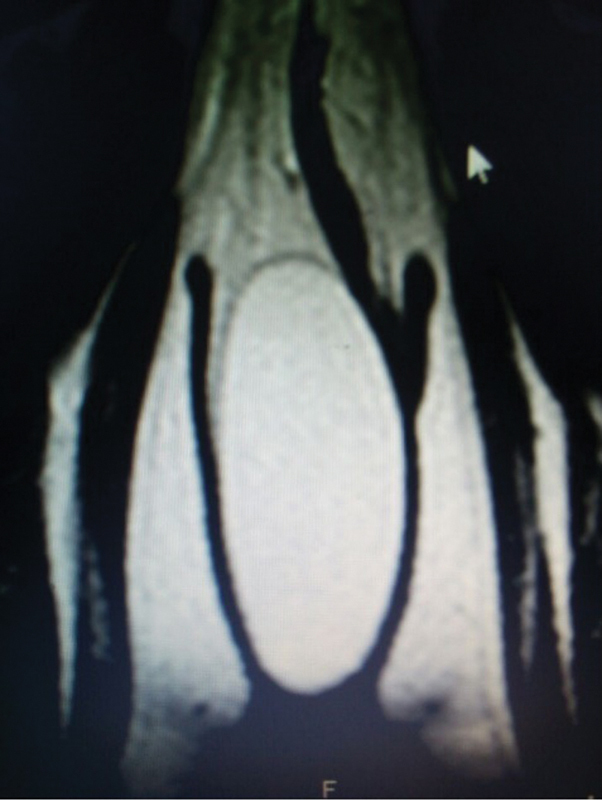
Magnetic resonance imaging scan showing oval soft tissue mass with well-defined capsule.
